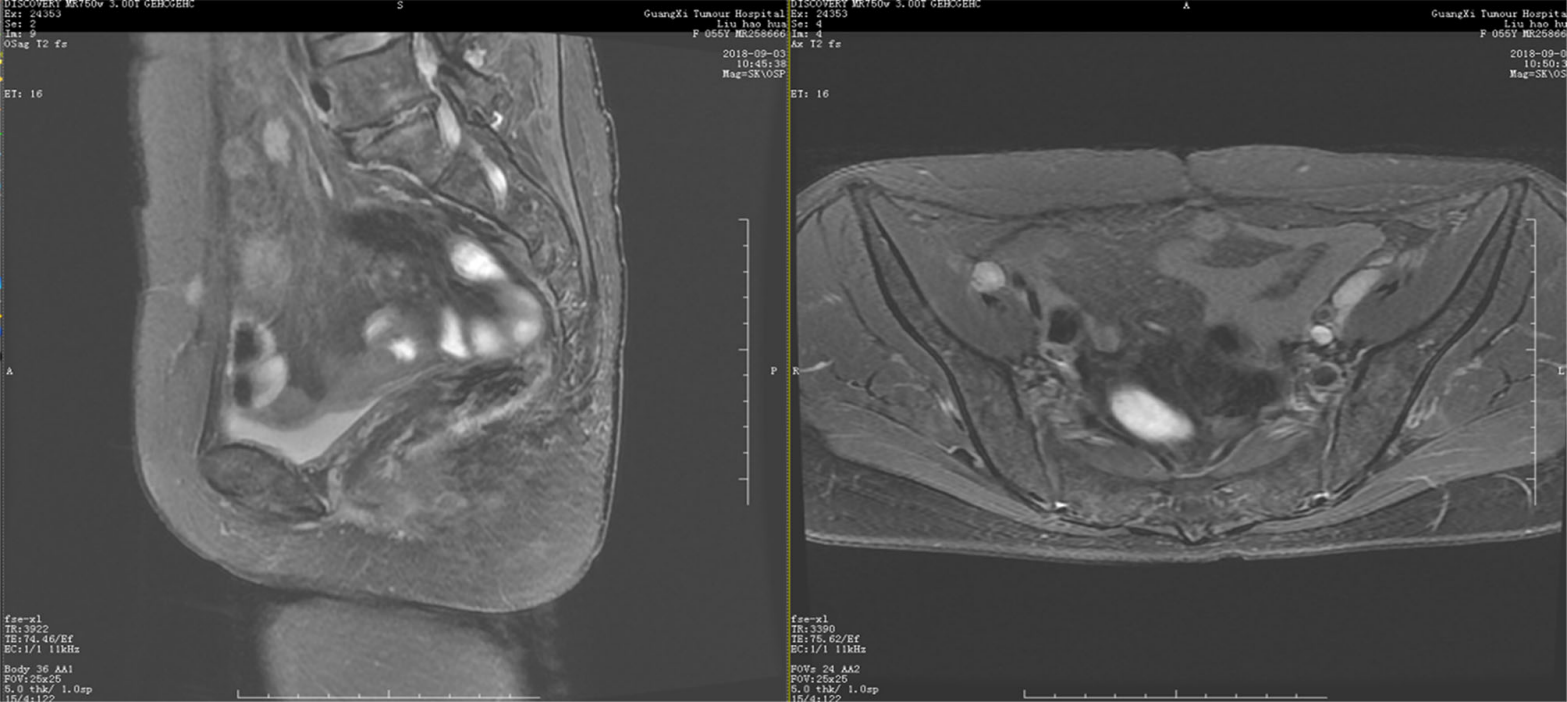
Pelvic MRI on September 3, 2018. Coronal view showing no new lesions after niraparib administration for one year.
